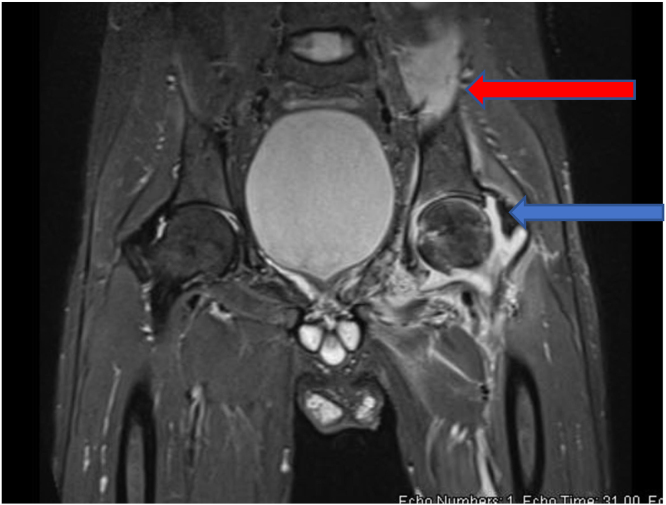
MRI of the left hip demonstrating synovial thickening (Blue) and inflammatory changes involving the surrounding muscles including the iliopsoas (Red). For interpretation of the references to color in this figure legend, the reader is referred to the web version of this article.
